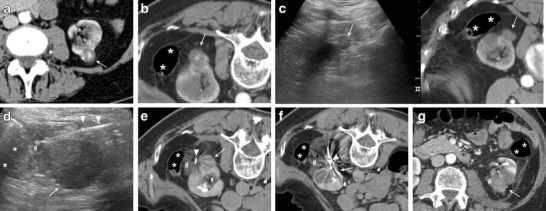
Microwave ablation of a left posterior renal cell carcinoma close to the colon with hydrodissection. a Contrast enhanced CT demonstrating a left posterior renal cell carcinoma (*white arrow*) to be treated with percutaneous thermal ablation. b Contrast enhanced CT performed the day of the procedure. To achieve a safe path to the tumour the patient is placed in prone position with external compression. In this position, the colon (*asterisks*) appears to be close to the tumour to be treated (*white arrow*). c fusion of contrast enhanced CT and real-time US allowed for the identification of the tumour to be treated with US (*white arrow = tumour; asterisks = colon*). d under US guidance a small spinal needle (*arrowheads*) is placed in between the tumour (*white arrow*) and colon (*asterisks*) and fluid (*hash*) is injected. e A CT scan performed after fluid (*hash*) injection confirmed the correct displacement of the colon (*asterisks*) from the target tumour (*white arrow*) (*arrowhead = spinal needle*). f CT scan demonstrating the insertion of the microwave antenna (*arrowhead*) into the renal tumour (*white arrow*) (*asterisks = colon; hash = injected fluid*). g Contrast-enhanced CT performed the day after treatment demonstrating the complete ablation of the renal tumour (*white arrow*)without complications (*asterisks = colon*)
